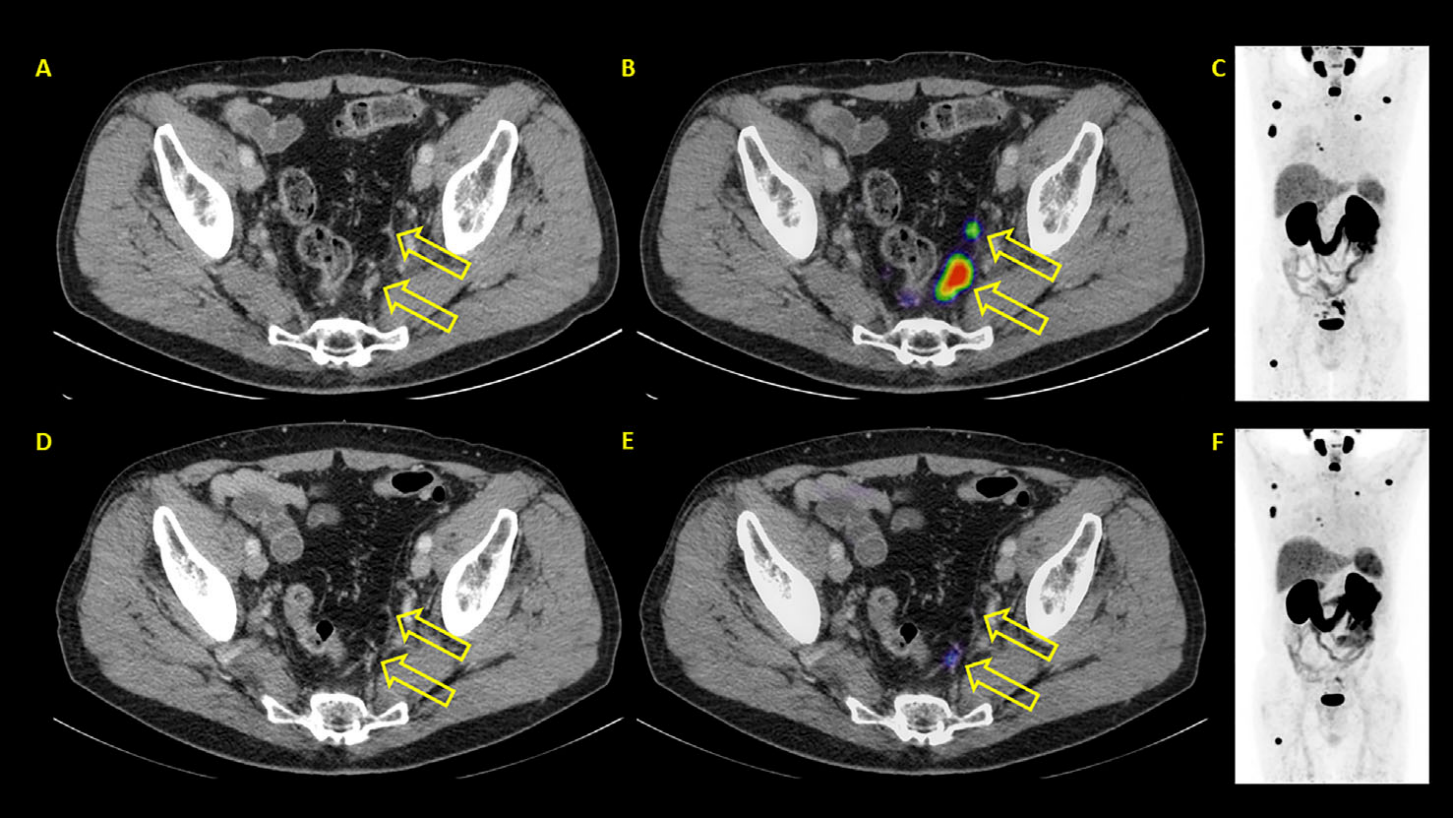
Example of a patient where PSA and PET showed discordant results. PSA showed progressive disease with PSA rising from 24.3 to 35.8 ng/ml. PSMA PET/CT showed partial remission. (A–C) represent baseline images whereas (D–F) represent interim PET/CT. (A, D): axial CT slices; (B, E): axial fused PET/CT images; (C, F): maximum intensity projection images.
